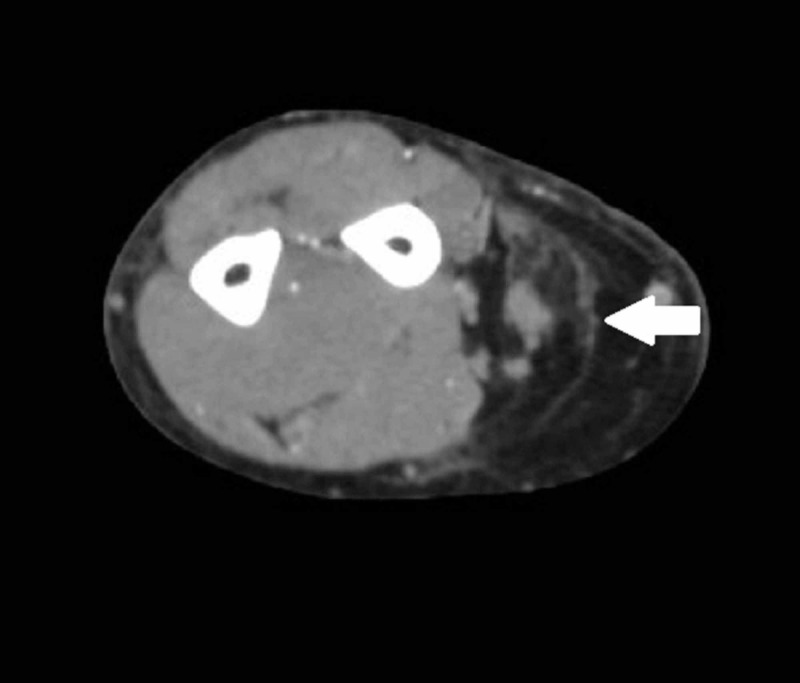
A 56-year-old male with a right forearm fat containing mass (thick white arrow) demonstrated on axial CT image, concerning for an atypical lipomatous tumor or liposarcoma.
